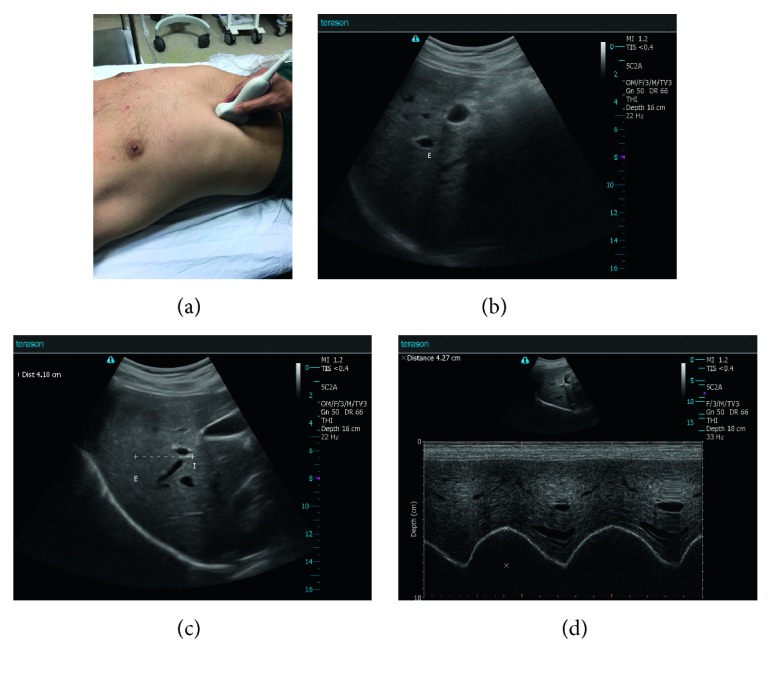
(a) The convex probe is positioned on the abdomen to examine the right diaphragmatic dome. The upward and downward movements of the right diaphragmatic dome were measured from the anterior position. The probe orientation should be longitudinal scan (right hemidiaphragm US method in B-mode and M-mode). (b and c) B-mode ultrasound evaluation of the craniocaudal displacement of the left branch of the portal vein in a patient with COPD. The position of the vessel was marked by the calliper during forced expiration and inspiration manoeuvres. The line shows displacement of the left branch of the portal vein during maximal inspiratory and expiratory breathing in the sagittal plane. The craniocaudal displacement of this branch was registered in millimetres. E marks at maximal end expiration, and I marks the lowest point at maximal inspiration. The distance between E and I is 41 mm (Ant B-Mode R). (d). M-mode scan of the right hemidiaphragmatic dome at maximal inspiration The first calliper was placed at the foot of the inspiration slope on the diaphragm echoic line and the second one at the apex of this slope for the deep breathing measurements (Ant M-Mode R: 42.7 mm).
